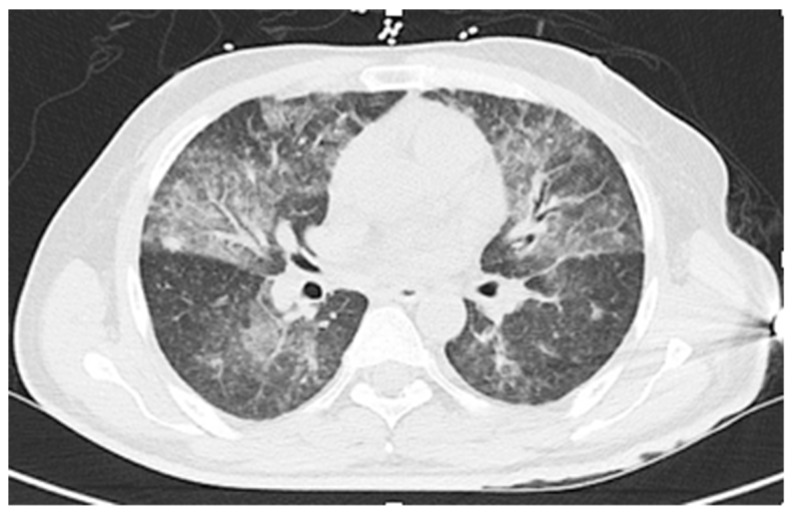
Case 2: CT of the chest showing extensive ground glass and patchy parenchymal opacities throughout the bilateral lungs; the differential diagnosis included opportunistic infections (pneumocytosis, cytomegalovirus), alveolar hemorrhage, and pulmonary edema.
